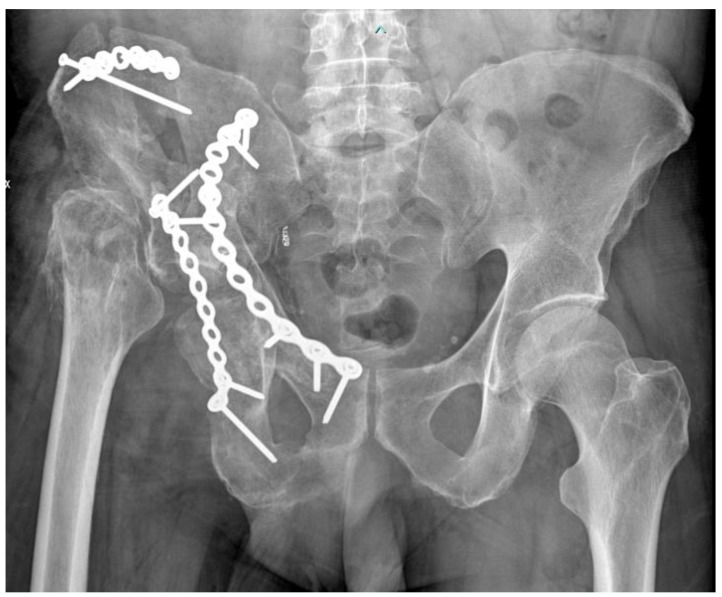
X-ray of the pelvis after the new osteosynthesis of the bicolumnar acetabular fracture.
